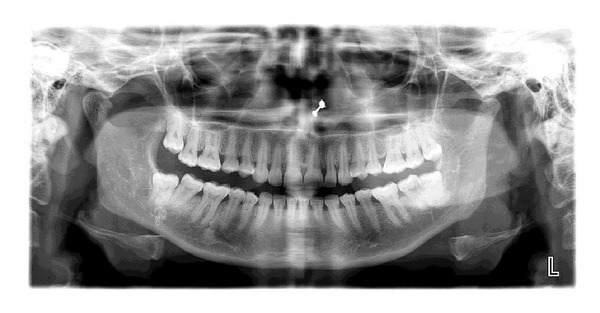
Panoramic radiograph revealing calcifications superimposed over the partially erupted lower right third molar.
